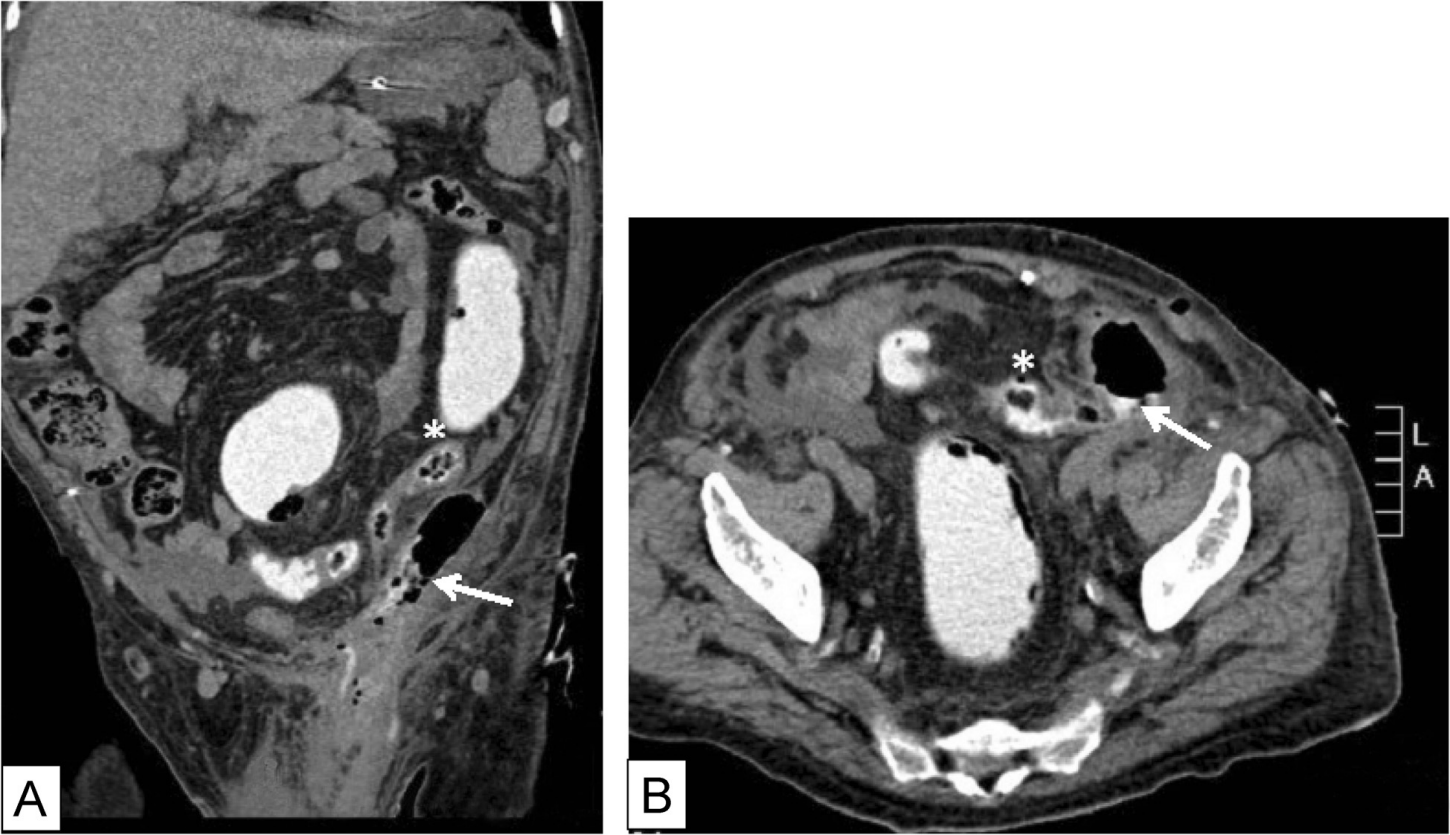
CT scan with rectal contrast revealing an enhanced preperitoneal space and fistulous tract(arrow), communicating between a sigmoid segment(*) and the internal ring of inguinal canal (a: coronal view; b: axial view)
